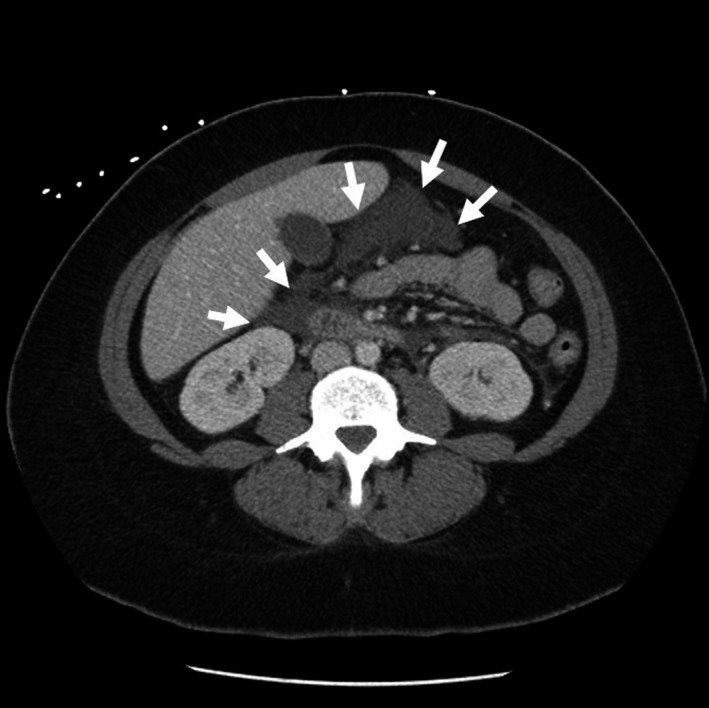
Computed tomography scan of the abdomen of a 37‐year‐old woman with nausea, vomiting, and diarrhea. The scan, on hospital day 1, revealed a peripancreatic fluid collection (arrows) compatible with acute pancreatitis.
